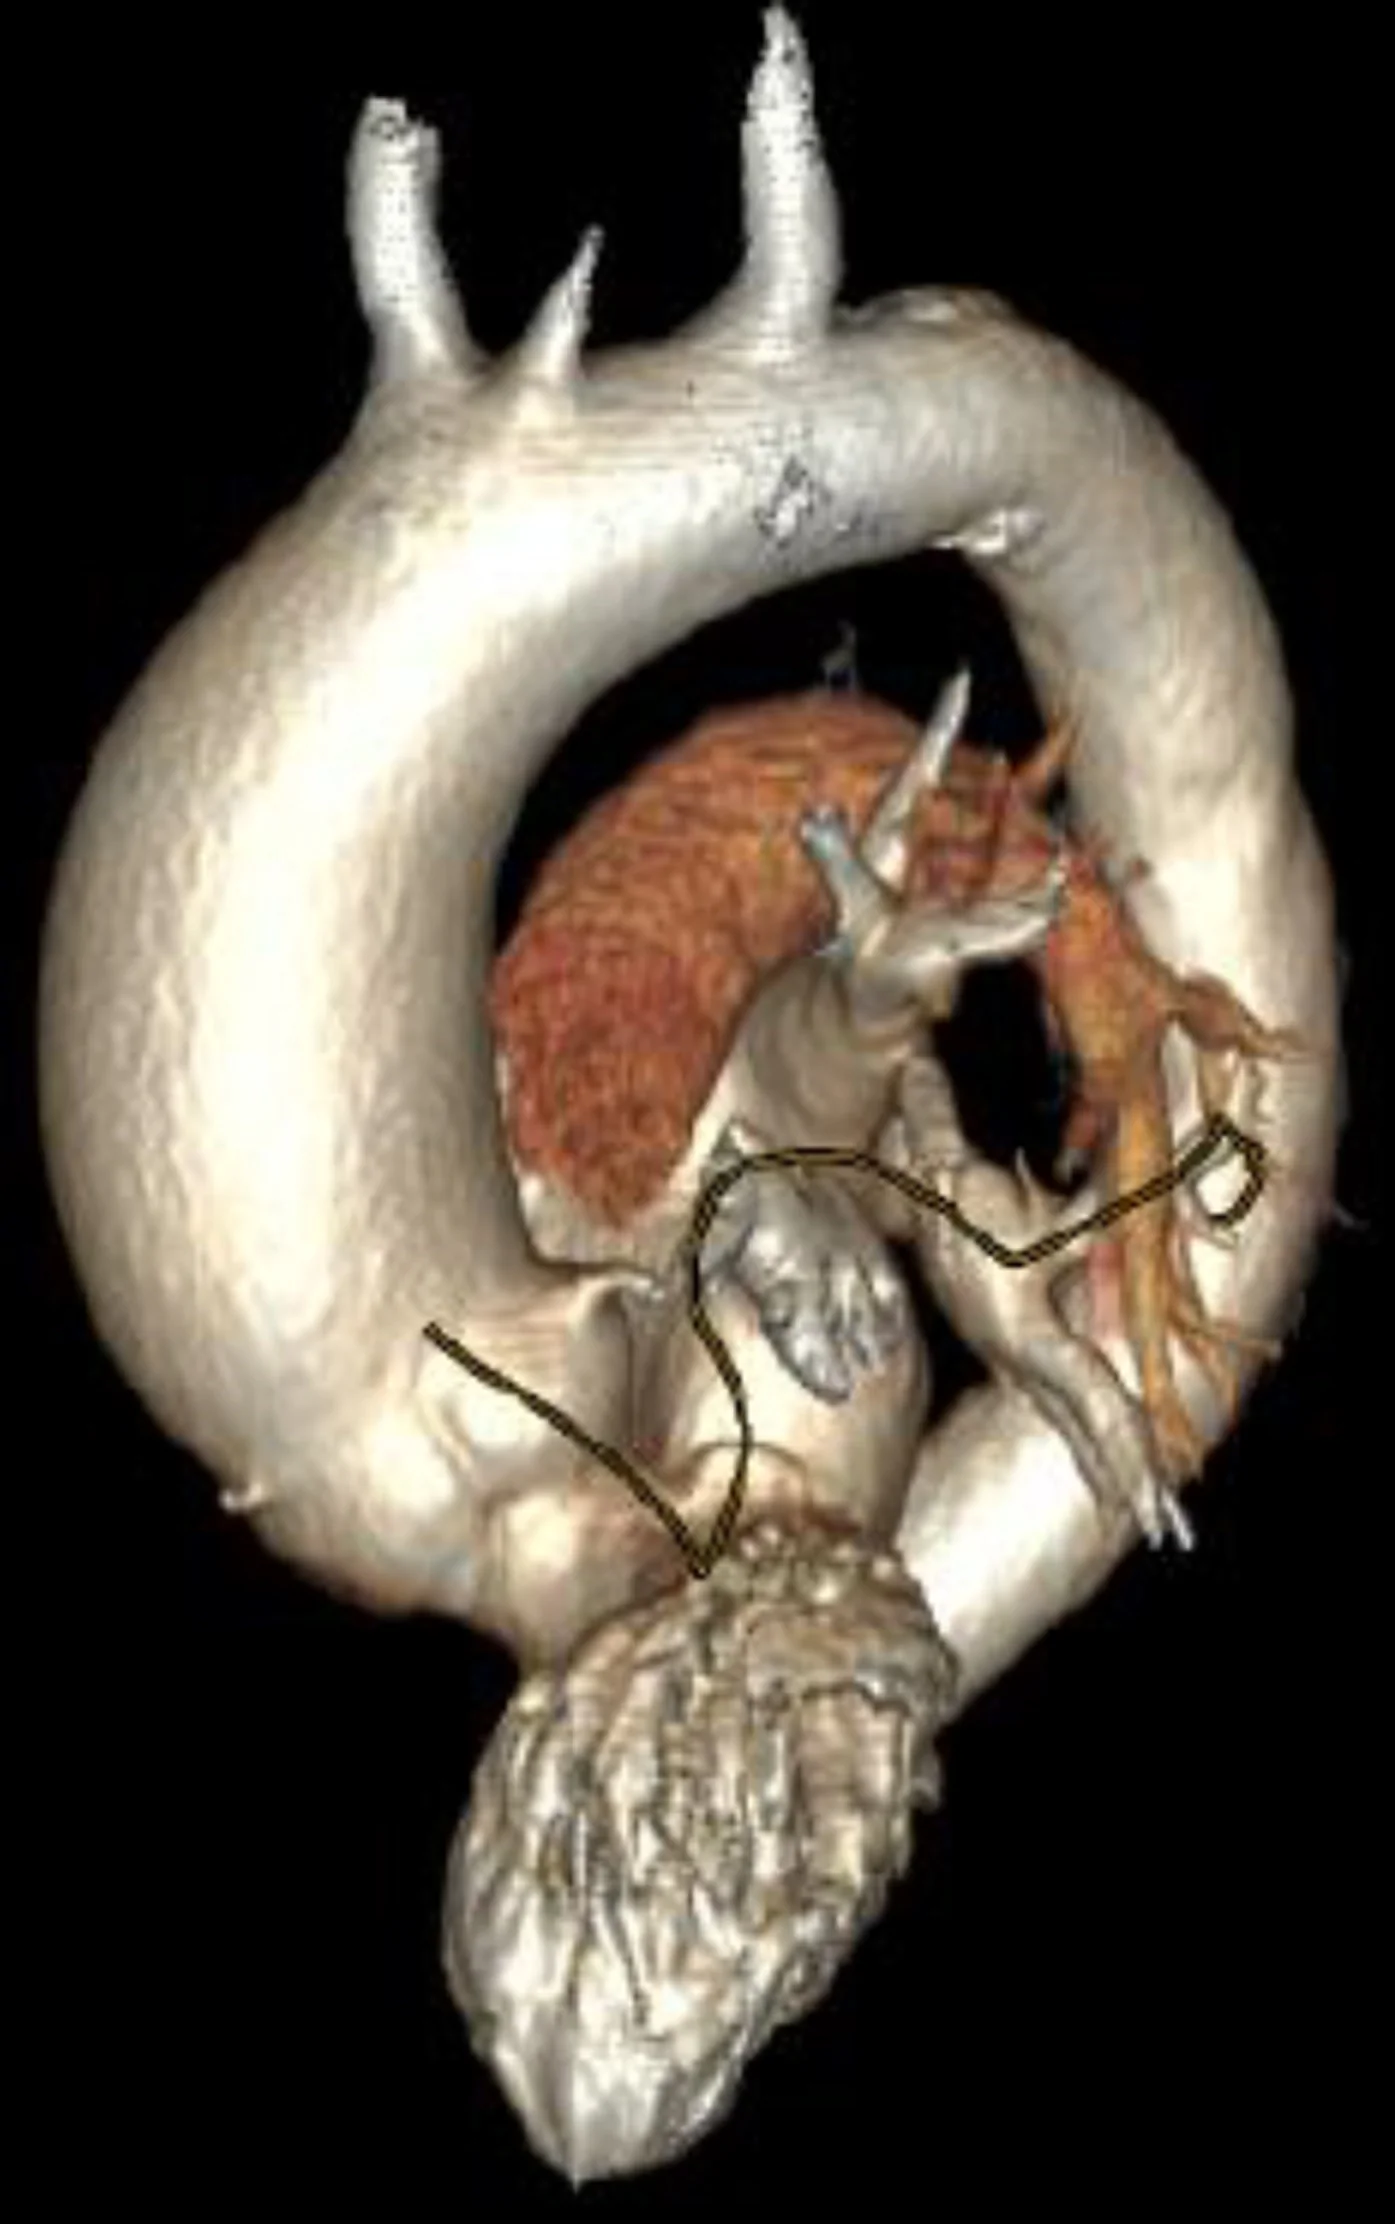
Computed tomography scan with a three-dimensional reconstruction of the thoracic aorta. Anulus 3.5 cm, sinus Valsalva 4.3 cm, sinutubular junction 4.0 cm, ascending aorta 5.2 cm, aortic arch after the origin of the left common carotid artery 3.0 cm, and descending aorta after the origin of the left subclavian artery 2.6 cm.
